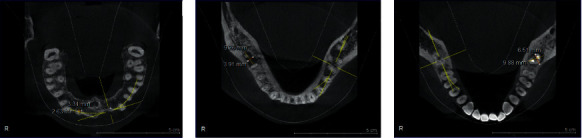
Different degrees of periapical lesions in patients with chronic apical periodontitis: (a) mild; (b) moderate; and (c) severe).
